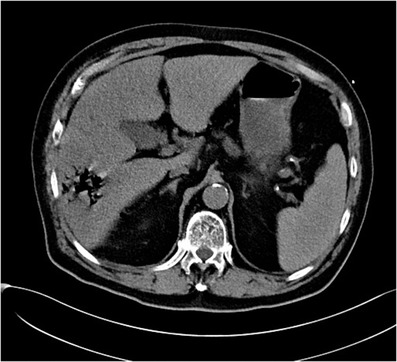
Computed tomography scan of the abdomen showing presence of a multiloculated liver abscess
